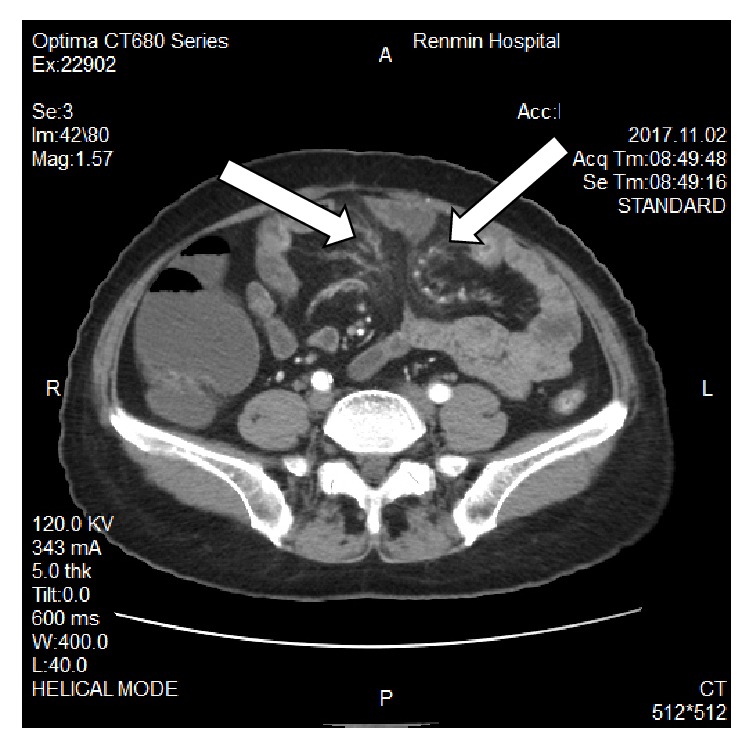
CT scan showing mesenteric edema, mesenteric fat blurred.
